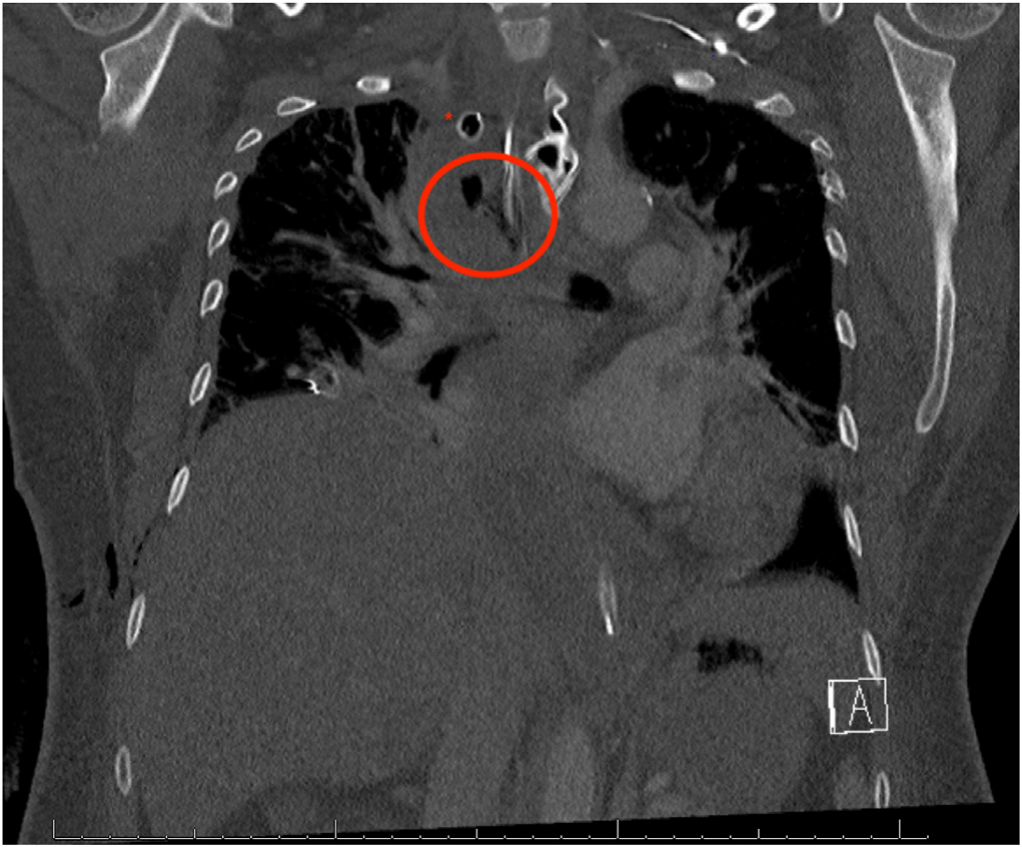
Coronal plane image from a CT scan before intervention by the thoracic surgery department demonstrating the esophageal intramucosal dissection (within the *red circle*) and anterior chest tube (indicated by the *red asterisk*).
